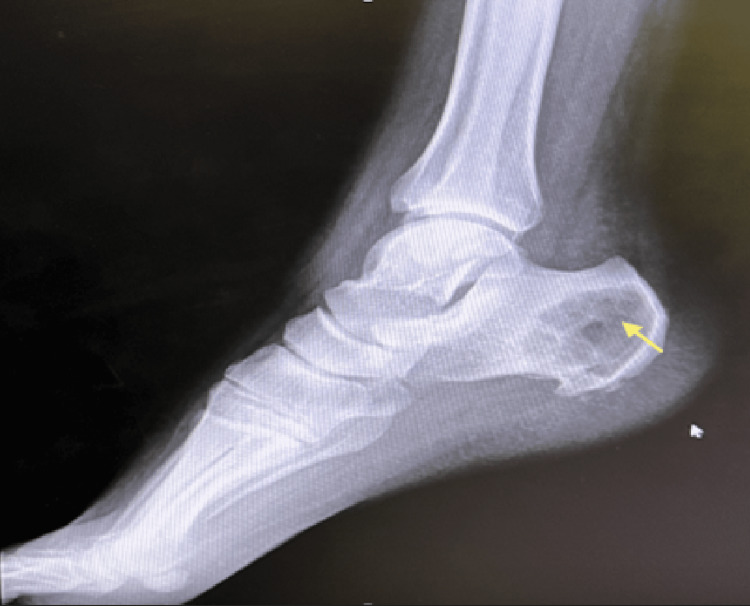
Initial X-ray of the right foot X-ray of the right foot showing a 4.3x3.5 cm lytic lesion with a soap bubble appearance (yellow arrow) at the posterior part of the right calcaneus with a wide transition zone.
